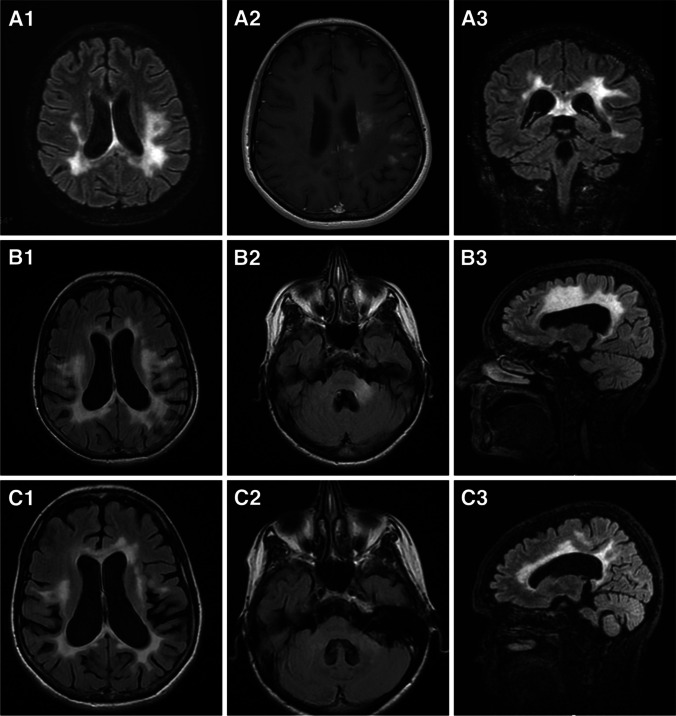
A MRI performed in 2019 showing the bilateral hyperintensity in the frontal and parietal white matter in the axial T2-FLAIR WI (A1), a focal and irregular post-contrast enhancement in the left periventricular white matter and parieto-occipital region in the axial T1-WI (A2) and the involvement of isthmus of corpus callous as well as the left frontal white matter in the coronal T2-FLAIR WI (A3). B MRI performed in January 2022 showing the extension of the bilateral hyperintensity involving the periventricular regions as well the parieto-occipital lobes in the axial T2-FLAIR WI (B1), the focal hyperintensity of the left middle cerebellar peduncle in the axial T2-FLAIR WI (B2) and the massive involvement of frontal deep and periventricular white matter in sagittal T2 FLAIR WI (B3). C MRI performed in October 2022 showing the reduction of hyperintensity in the axial T2 FLAIR WI (C1), the partial reduction of the hyperintensity in the left middle cerebellar peduncle in the axial T2-FLAIR WI (C2) and the partial regression of the hyperintensity in the right middle superior frontal gyrus in the sagittal T2-FLAIR WI (C3). In September 2023, the MRI was repeated and resulted stable in comparison to that performed in October 2022
